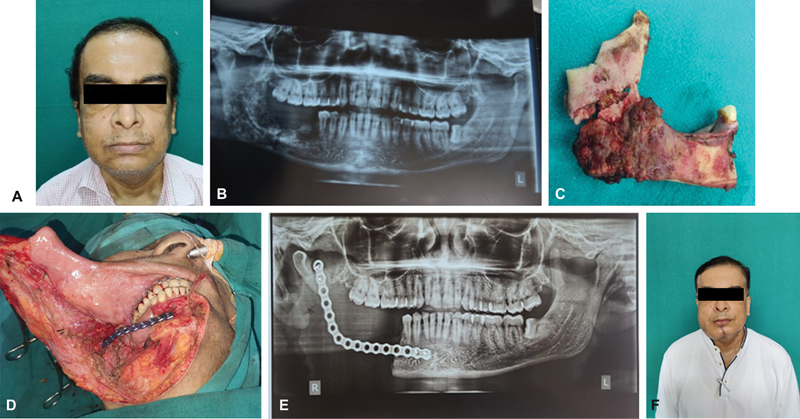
(A) Preoperative photograph with mild swelling evident over left lower jaw. (B) Orthopantomogram showing honeycomb appearance and osteomyelitis of the right mandible. (C) Blackish discoloration of the bone with thinned-out cortex at the junction of body and ramus. (D) Defect after segmental mandibulectomy and reconstruction plate in situ. (E) Orthopantomogram showing mandible plate in situ. (F) One month follow-up after mandible reconstruction with free fibula osseous flap.
